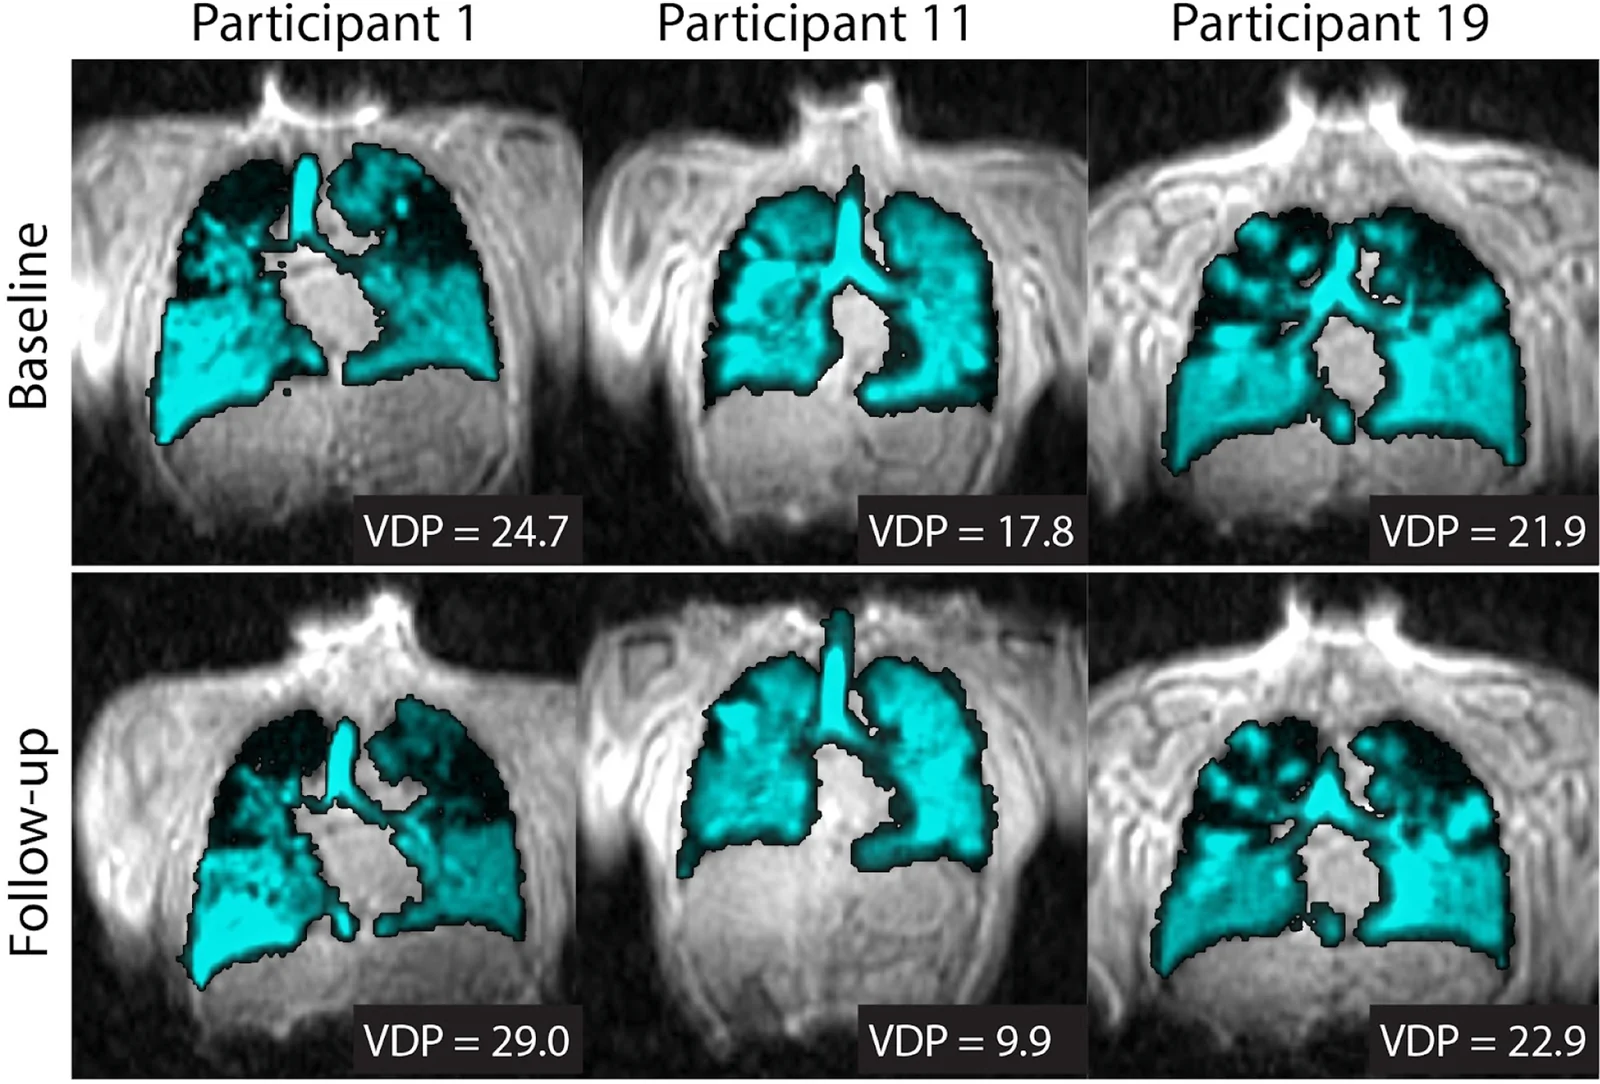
Representative hyperpolarized xenon-129 MRI ventilation images for 3 participants at baseline and follow-up. Although some participants had significant changes to ventilation (eg, participant 11), most participants had minimal change in ventilation with similar VDP and size/location of ventilation defects across time points. VDP = ventilation defect percent.
